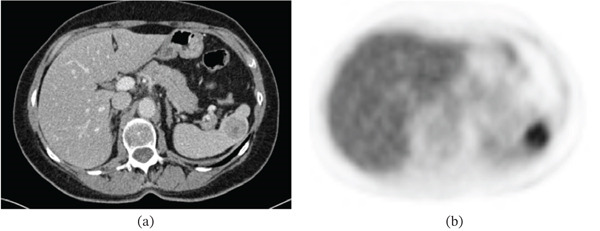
(a) CT and (b) PET images of the abdomen revealing a solitary splenic lesion. The lesion is hypodense on CT and hypermetabolic on PET. It was later confirmed as an isolated splenic metastasis from lung adenocarcinoma.
